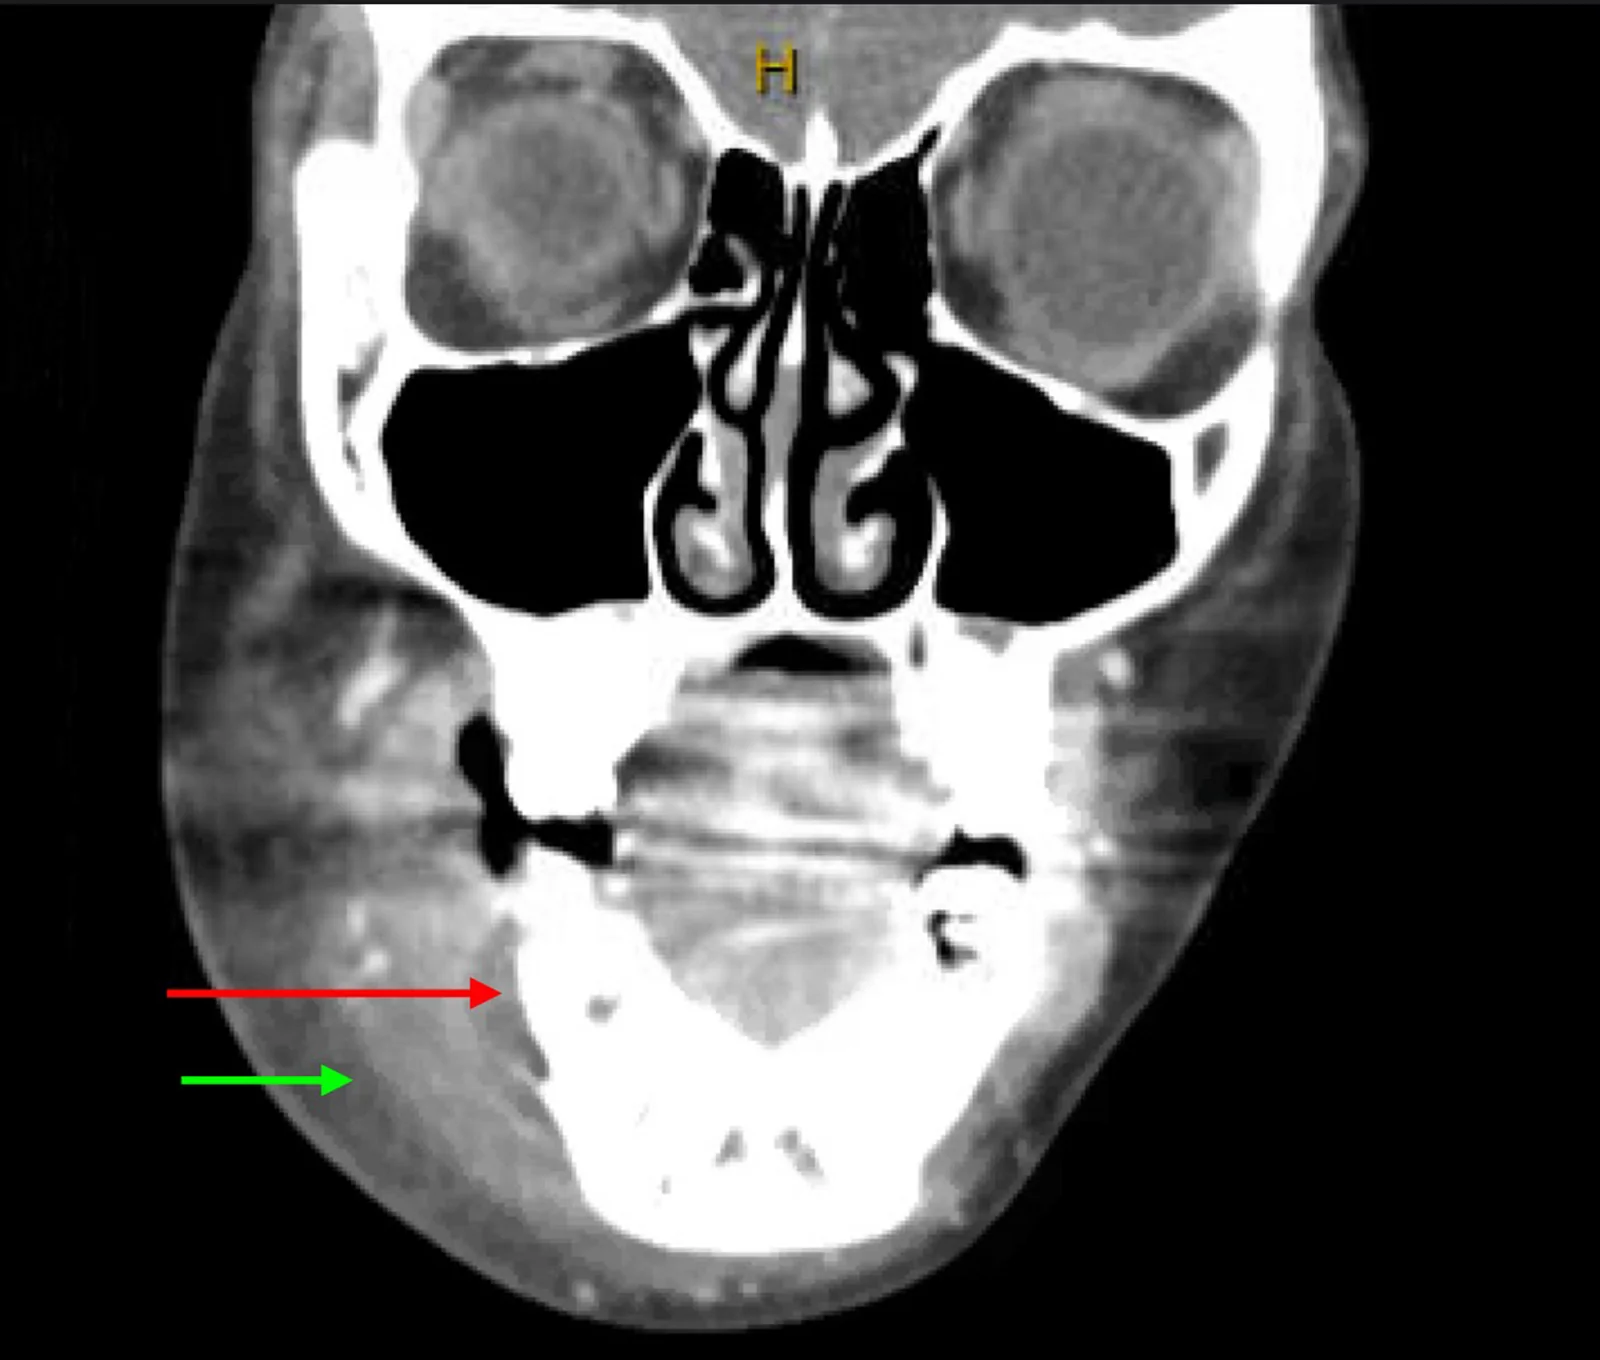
Coronal view of CT scan—soft tissue of the neck. The red arrow denotes the subperiosteal abscess, and the green arrow denotes subcutaneous reticulation and skin thickening compatible with right facial cellulitis and swelling. CT, computed tomography.
